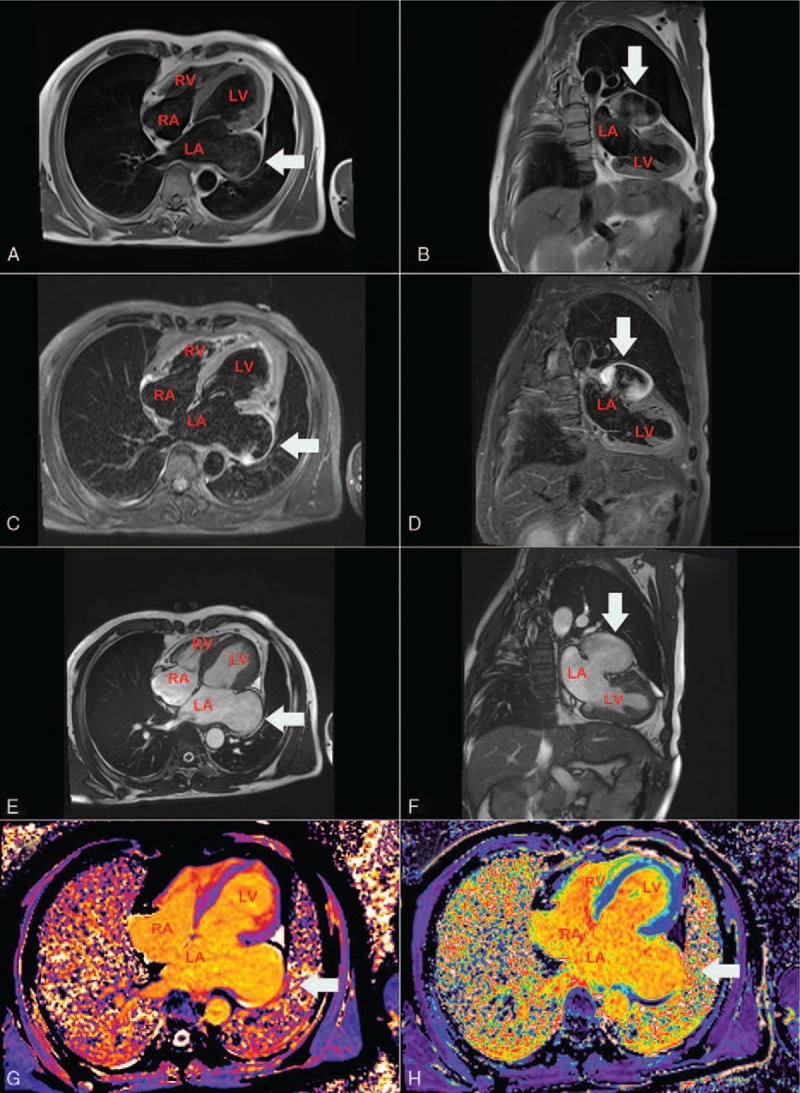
MRI. Axial spin-echo T1-weighted (E) and Coronal spin-echo T1-weighted image (F) showing the LAA aneurysm with no evidence of thrombus. LA = left atrium, LV = left ventricle, MRI = magnetic resonance imaging, RA = right atrium, RV = right ventricle.
